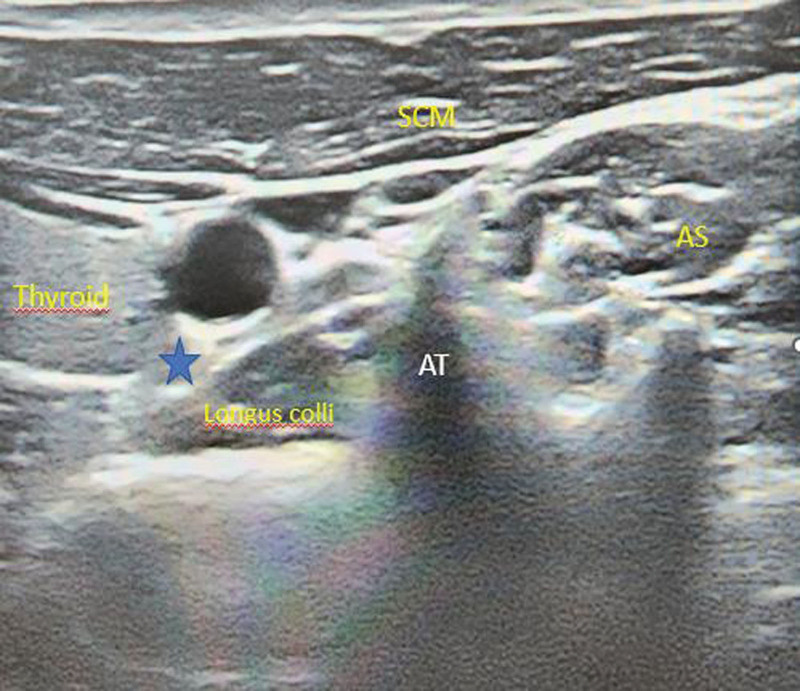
Ultrasound-guided stellate ganglion blockade. ASM = anterior scalene muscle, AT = anterior tubercule, CA = carotid artery, SCM = sternocleidomastoid muscle. Blue star: Target point.
